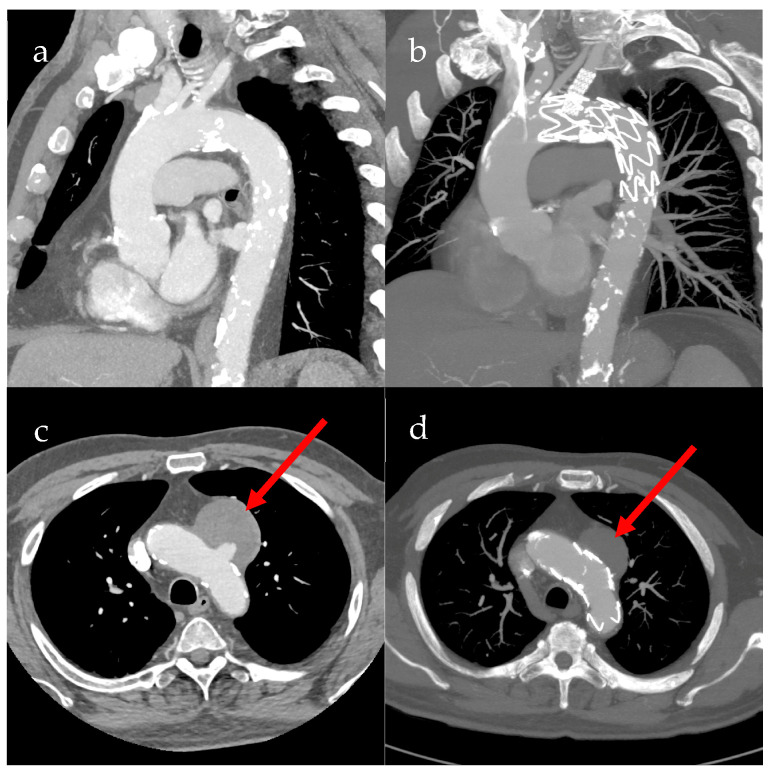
Pre- and postoperative CT scans of in situ fenestrated thoracic endovascular aortic repair (isfTEVAR). (a) Sagittal preoperative CT image of the aortic arch; (b) sagittal postoperative CT image of the aortic arch; (c) transverse preoperative CT image of the aortic arch, red arrow showing perfused penetrating aortic ulcer (PAU); (d) transverse postoperative CT image of the aortic arch, with red arrow showing non-perfused PAU.
